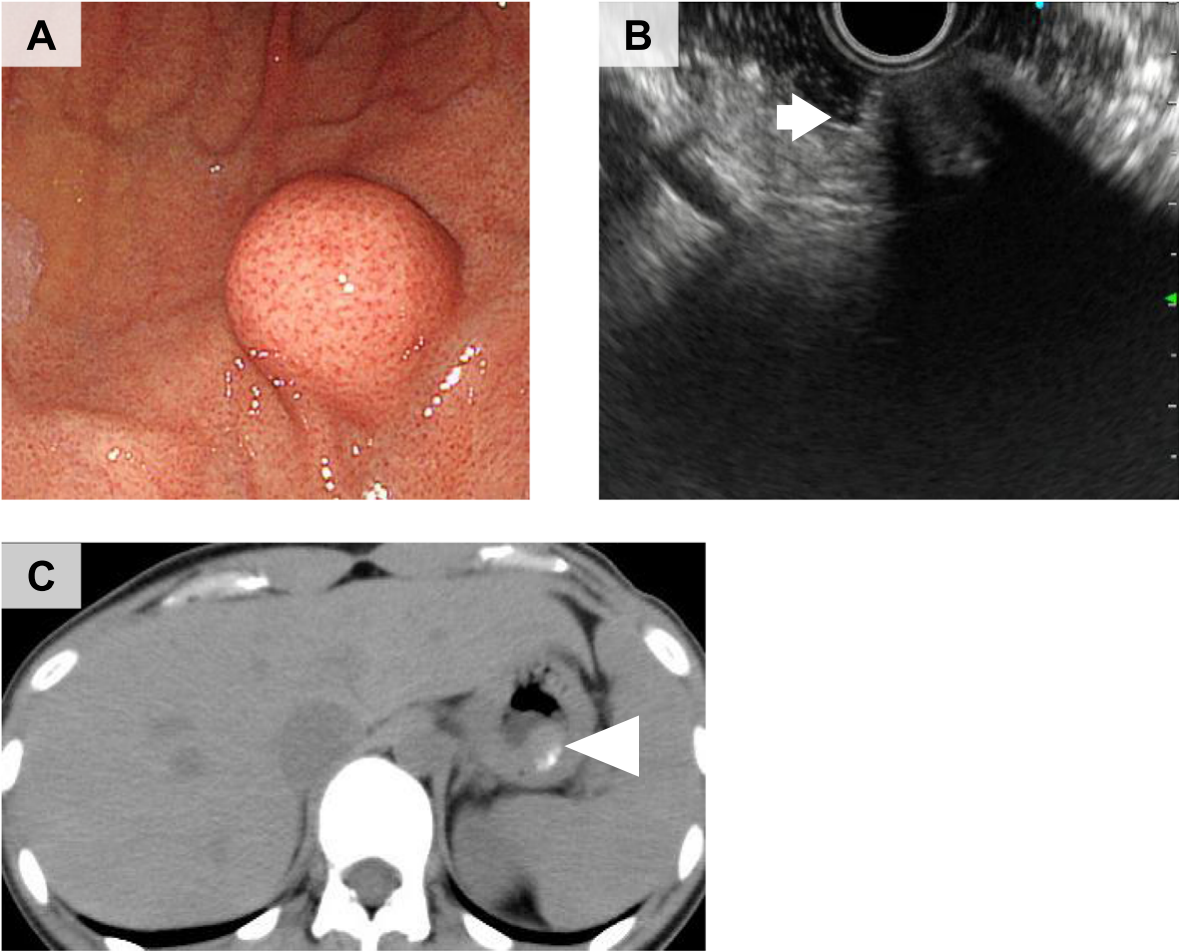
Upper gastric endoscopy showed a submucosal tumor in the gastric body (a). Endoscopic ultrasonography demonstrated a heterogeneous hypoechoic lesion with a shadow (b, arrow), consistent with calcification. Computed tomography showed calcifying gastric mucosal tumor (c, arrowhead)
